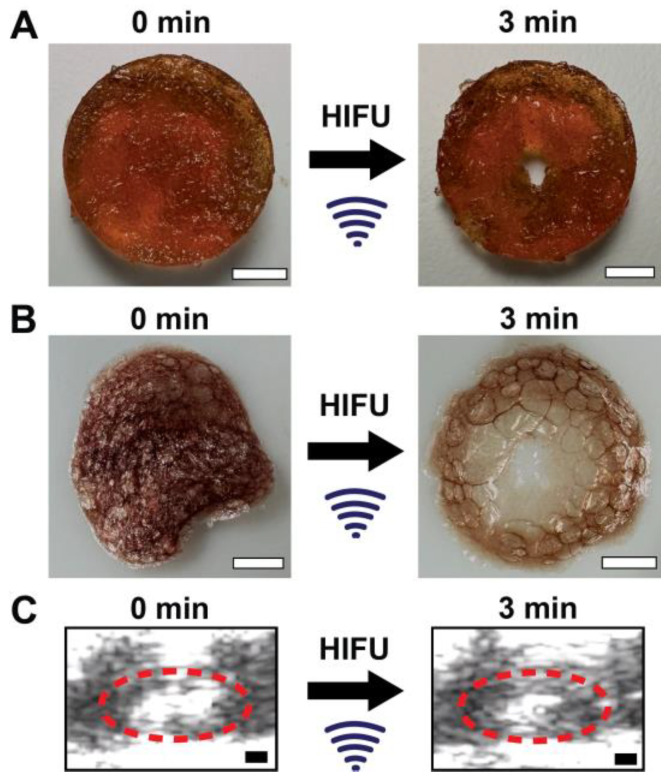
(A) An 8 mm PEG-FDA-4 hydrogel with no focused ultrasound exposure (left) and a hydrogel with 3 min of fUS exposure at 1.5 MHz with 20 ms repeated pulses at 1 Hz and *p*+ = 33 MPa and *p*- = 15 MPa (right) (scale bar = 2 mm). (B) The samples from A after being dried in a desiccator to visualize the crosslinking matrices (scale bar = 2 mm). (C) Real-time ultrasound imaging of PEG-FDA-4 before (left) and after 3 min of focused ultrasound (right), where the white space inside the red circle is the entirety of the PEG hydrogel (scale bar = 2 mm).
